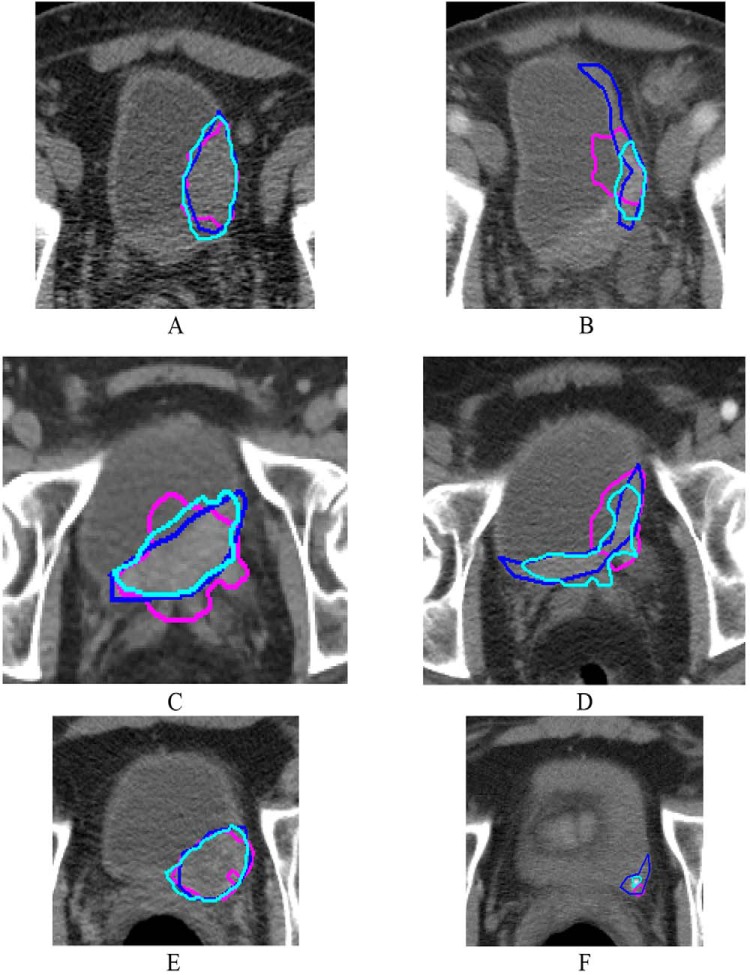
Examples of segmentations of bladder tumors in pre-treatment (A, C, E) and post-treatment (B, D, F) CT scans. The DL-CNN segmentation is shown in light blue. The auto-initialized cascaded level sets (AI-CALS) segmentation is shown in pink. The hand-outline is shown in dark blue. DL-CNN segmentation with AI-CALS segmentation and hand-outline for the cancer are shown in Figure 1 (A). Both computer methods segmented the lesion reasonably. The cancer lesion shrunk in size because of treatment, and became a part of the bladder wall (B). The DL-CNN undersegmented the cancer, not extending enough into the bladder wall. AI-CALS oversegmented the lesion, leaking into the bladder. The DL-CNN segmentation outlined the cancer relatively accurately, whereas the AI-CALS segmentation leaked (C). In this post-treatment scan, the cancer along the bladder wall was reasonably segmented by DL-CNN, whereas the AI-CALS was unable to follow the shape and leaked into the bladder (D). Both DL-CNN and AI-CALS segmented the bladder cancer reasonably well, but the AI-CALS slightly undersegmented the cancer (E). The bladder cancer responded to treatment, and thus, had shrunk considerably, making the segmentation difficult (F). Both the DL-CNN and the AI-CALS undersegmented the lesion.
